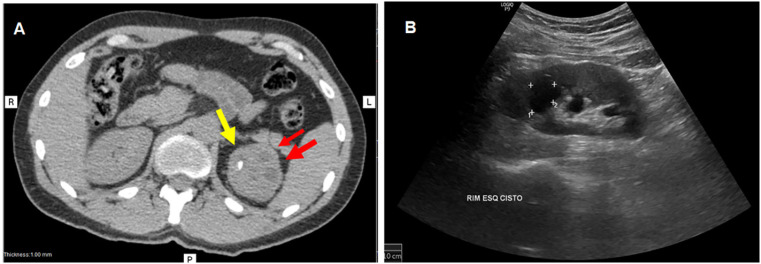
(A) Abdominal CT showing hypodense nodules in the left kidney, a larger one measuring 2.8 × 2.1 cm in the upper third (red arrows) and a smaller nodule measuring 0.7 × 0.3 cm (yellow arrow). A small ectasia of the pyelocaliceal system in this kidney is also observed; (B) Urinary ultrasound showing a cyst in the upper third of the left kidney with parietal calcification and a mixed solid-cystic nodule in the same region.
